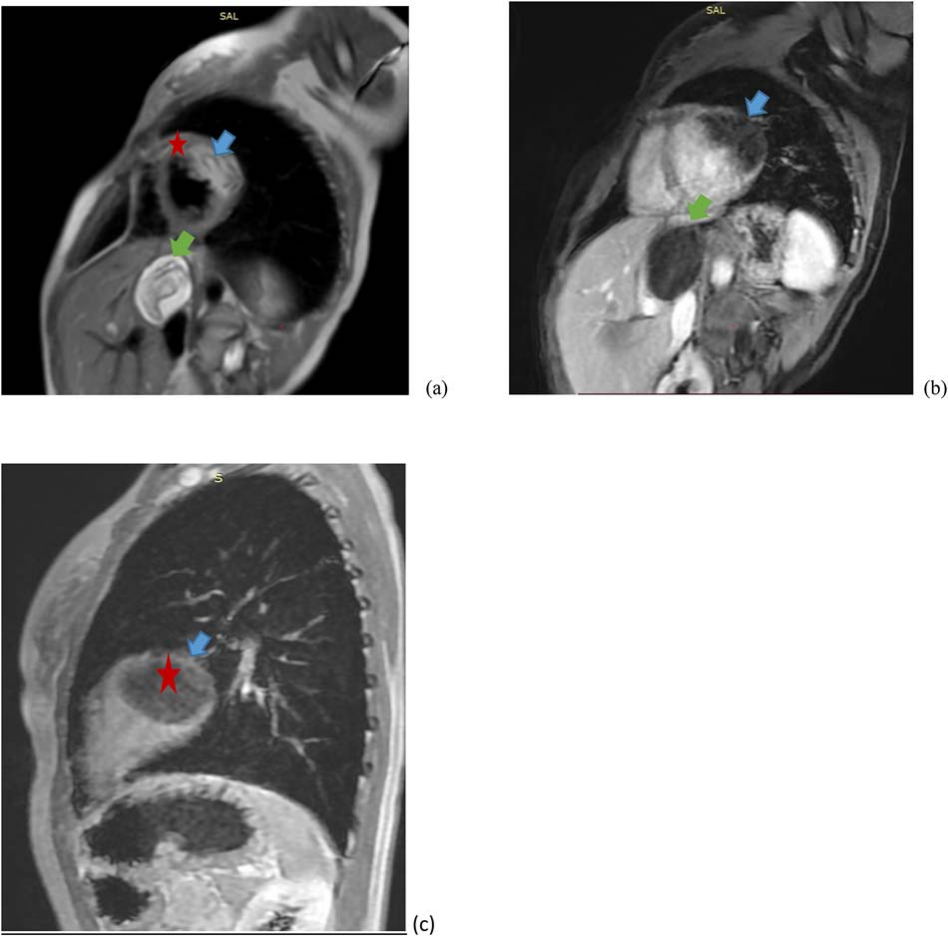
A 13 year-old femal patient presented with a chief complaint of cough, shortness of breath and palpitations. The cyst that includes membranes, is hyperintense on T2-weighted images (A) and mildly hypointense on T1-weighted axial (B and C) images (star) located in left ventricle posterior wall myocardium is.
